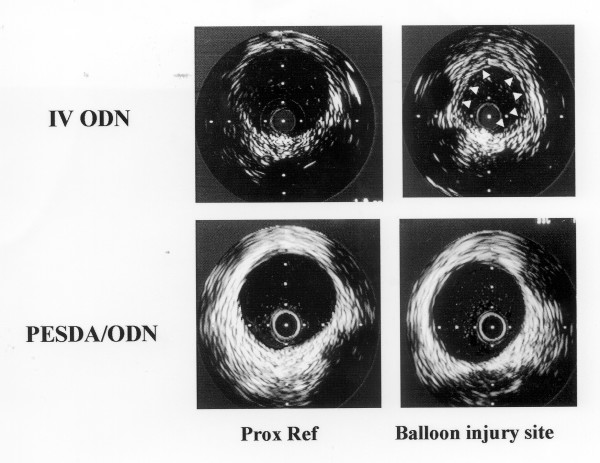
Intravascular ultrasound examples of the proximal reference site and balloon injury site 30 days after the angioplasty. Note that there was both greater intimal thickening (arrows) in the vessel treated with intravenous antisense alone, and a reduction in lumen size when compared to the proximal reference segment. The balloon injury site of the vessel treated with intravenous antisense plus PESDA and 20 kHz transcutaneous ultrasound did not exhibit any reduction in lumen area or visually evident plaque. (Reprinted with permission from Porter TR, Hiser WL, Kricsfeld D, Deligonul U, Xie F, Iversen P et al: Inhibition of carotid artery neointimal formation with intravenous microbubbles. Ultrasound Med Biol 2001, 27:259-265).
